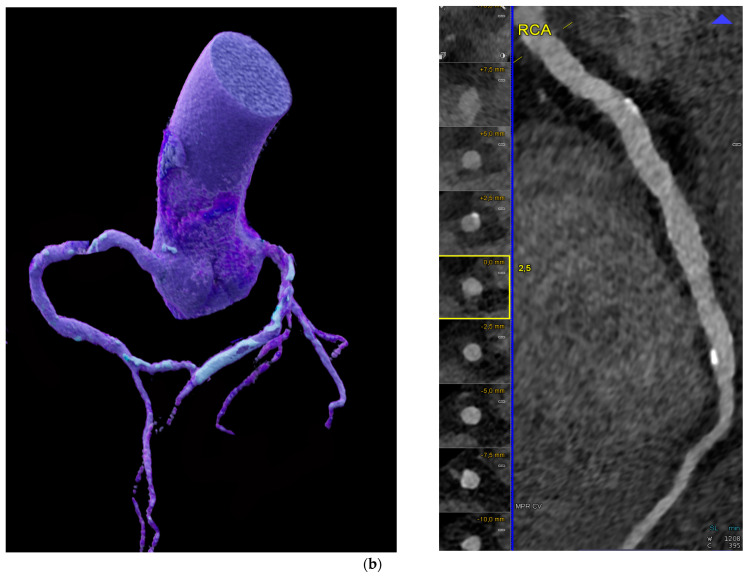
(a). Imaging of coronary stents by conventional energy-integrating detector (EID)-CT: Multiple left anterior descending (LAD) coronary stents with inadequate in-stent lumen visualization of the distal LAD and circumflex artery (CX) stent due to artifacts. Left: volume-rendering technique (VRT) and right: curved multiplanar reformations (cMRP) of the LAD and CX. (b). Photon-counting detector CT (PCD-CT): Curved multiplanar reformation (cMRP) (left) shows calcified right coronary artery (RCA) in a 76-year-old male patient with a high calcium load, but reduced blooming artefacts with less than 50% stenosis. Stent in the mid-left anterior descending (LAD) proximal coronary artery (VRT, right panel) and heavy vessel wall calcification. VRT = volume-rendering technique.
